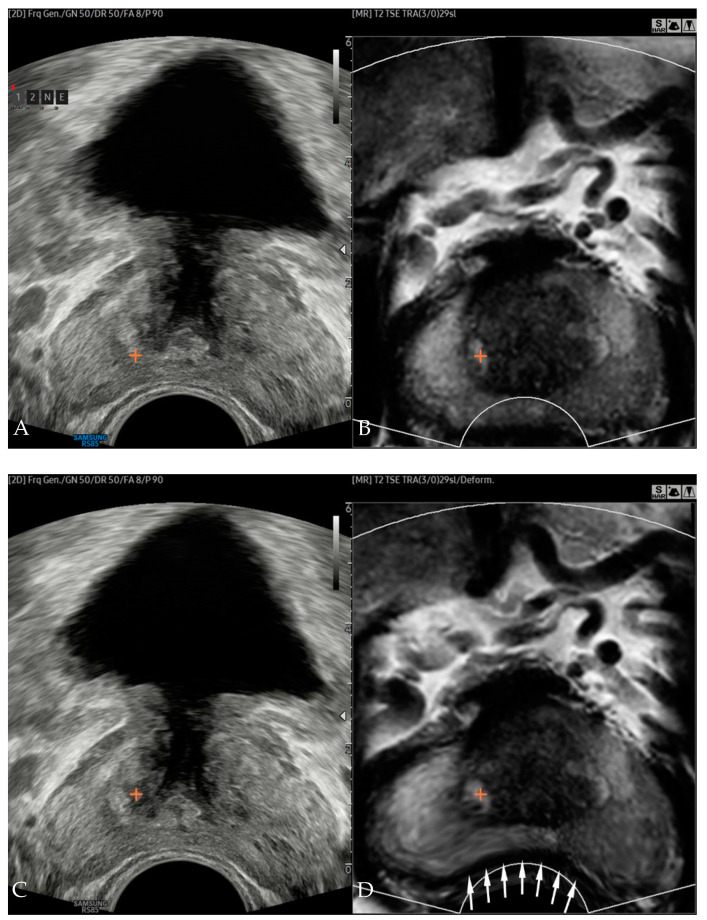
Side-by-side display of US (A) and MRI (B) of a 65-year-old male after rigid registration. Cross-mark drawn on MRI transferred to the corresponding point on US. Similar to the US (C) with a transrectal probe inserted, MRI (D) demonstrated concavely deformed posterior margin (arrows) after real-time corrected nonrigid registration. Internal image features of MRI were also deformed. Cross-mark selected on the deformed MRI also moved to its new corresponding point in the US.
